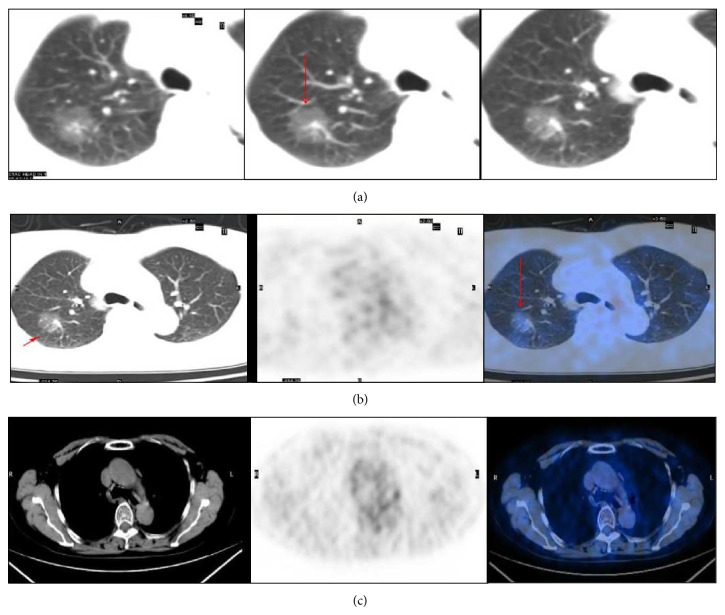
A 75-year-old female with an adenocarcinoma lesion with BAC features (red arrow). (a) Axial thin section CT images revealed a 2.8 cm pure ground-glass opacity in the right upper lobe. (b, c) ^18^F-FDG PET/CT was negative for the lesion, and the maximum standardized uptake value = 0.8.
